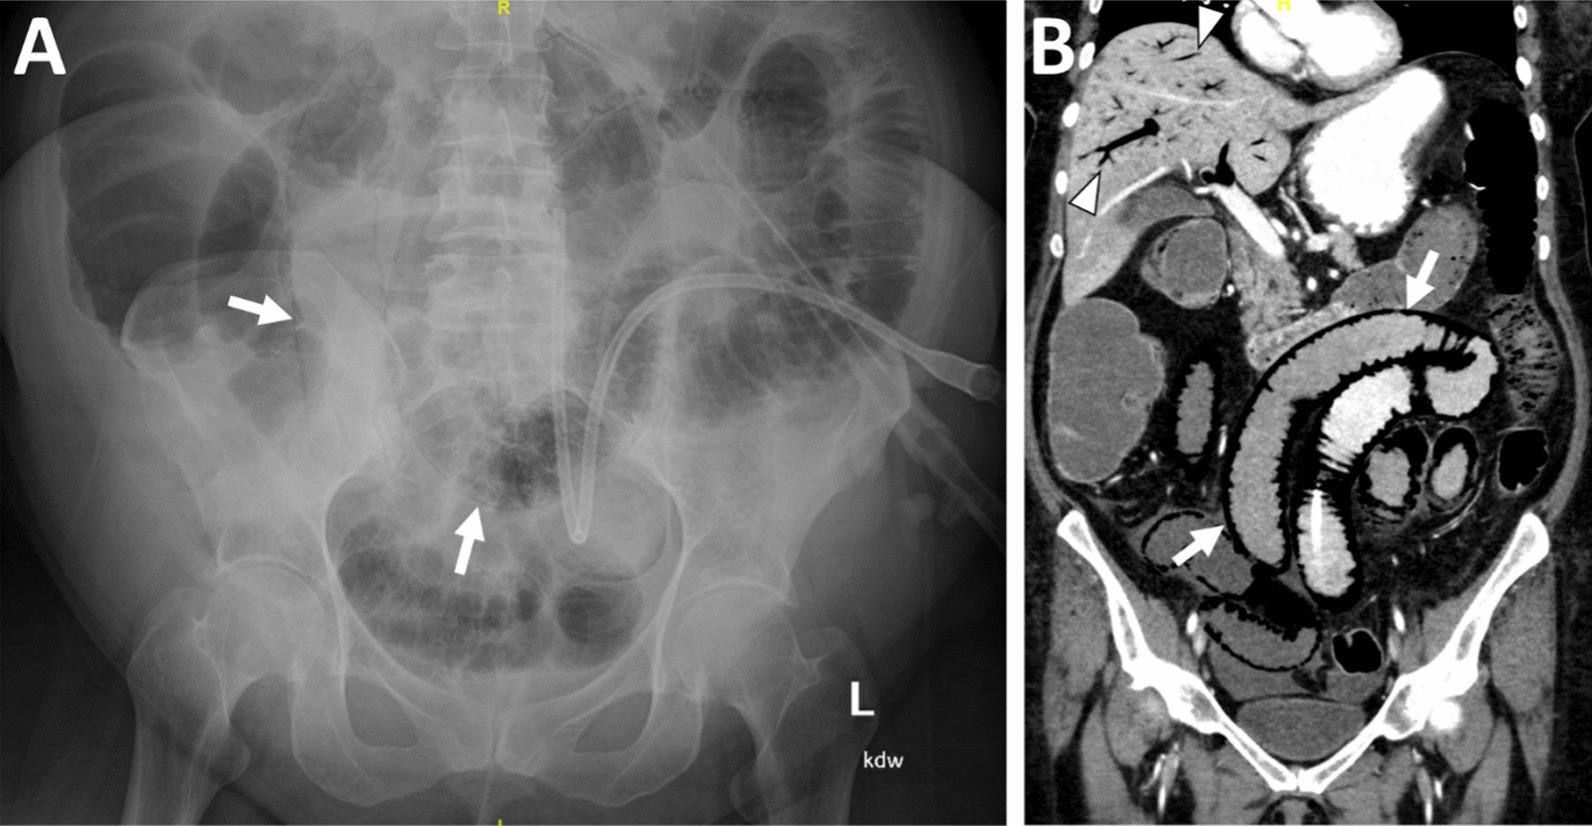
Abdominal X-ray A and CT B showing pneumatosis intestinalis (arrow) and portal venous gas (arrowhead)
